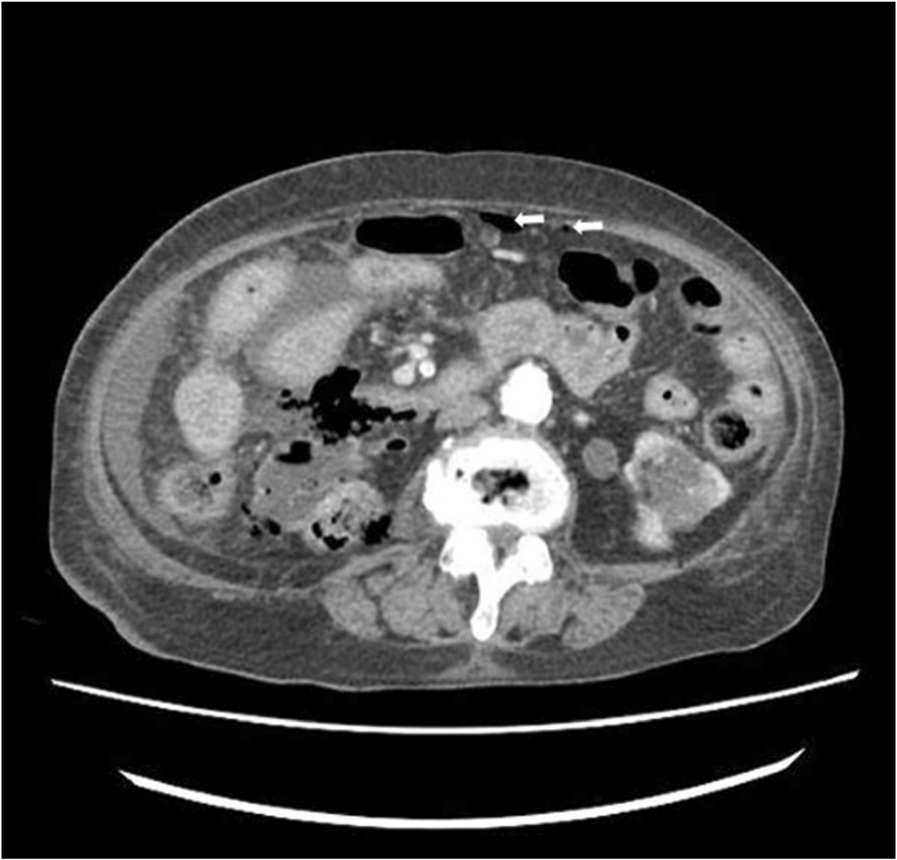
Abdominal CT finding. Axial view showing gas in the right renal parenchyme and perirenal space and intraperitoneal air (arrows)
